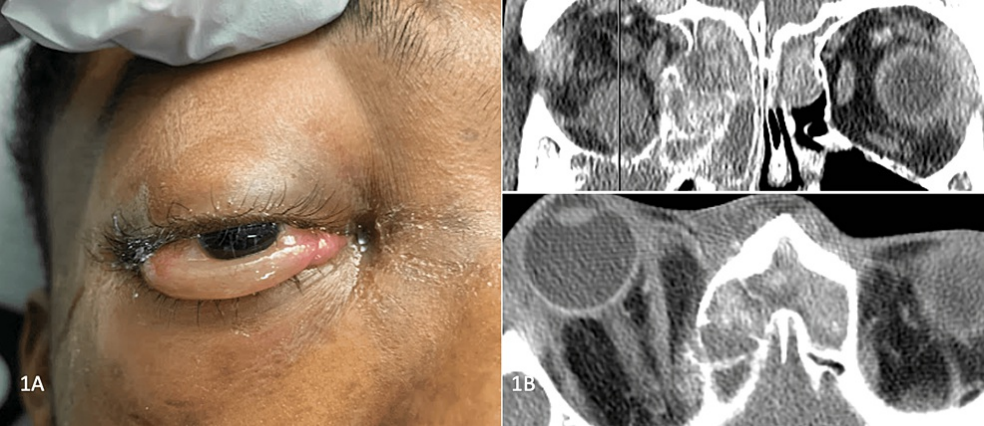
Initial presentation - 1A. Photograph of the right eye with chemosis and proptosis. 1B. Initial CT scan coronal and axial views.
